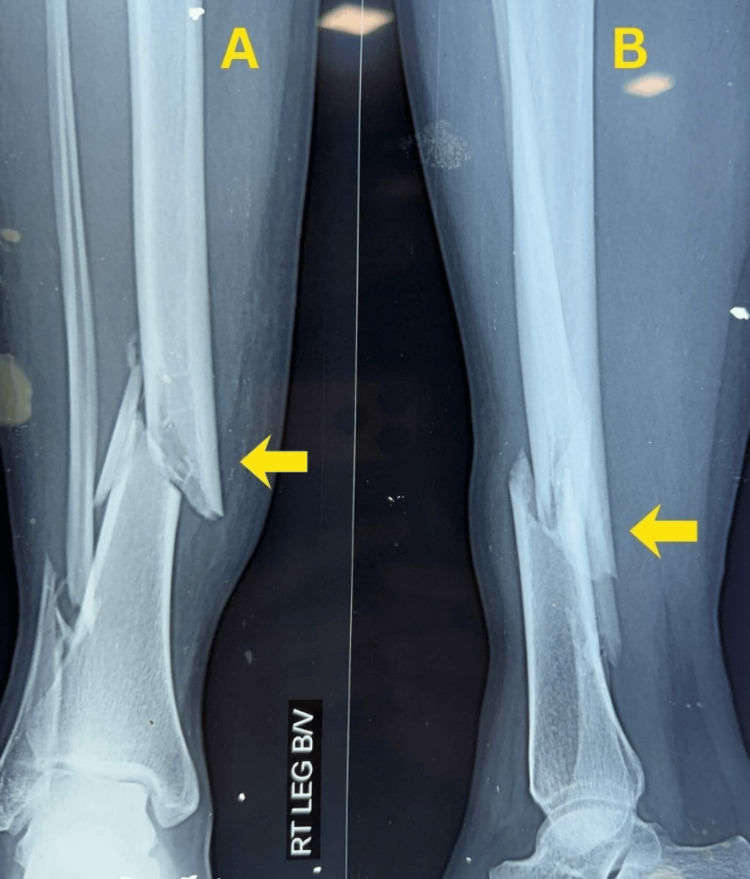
Preoperative radiographs of Case 3. (A) Anteroposterior view of the right distal tibia showing an extra-articular distal tibial fracture with associated fibular fracture. (B) Lateral view demonstrating fracture configuration, displacement, and distal tibial involvement.
